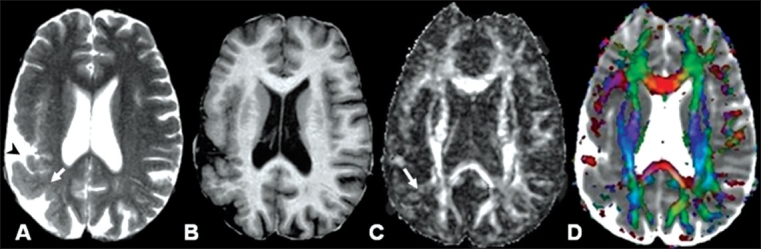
A 43-year-old man with a history of generalized seizures for the last 21 years. T2W axial image (A) through the lateral ventricles shows an abnormally thick cortex (arrow) with a bumpy appearance at the surface (arrowhead) and at the cortex-white matter junction in the right parietooccipital region. The abnormality is more prominently visible on the phase-sensitive T1 fluid-attenuating inversion recovery (FLAIR) axial image (B). The FA map (C) shows a diminished value (arrow) in the subcortical white matter subjacent to the polymicrogyric cortex, which is clearer on the color-coded FA modulated with MD map (D)
